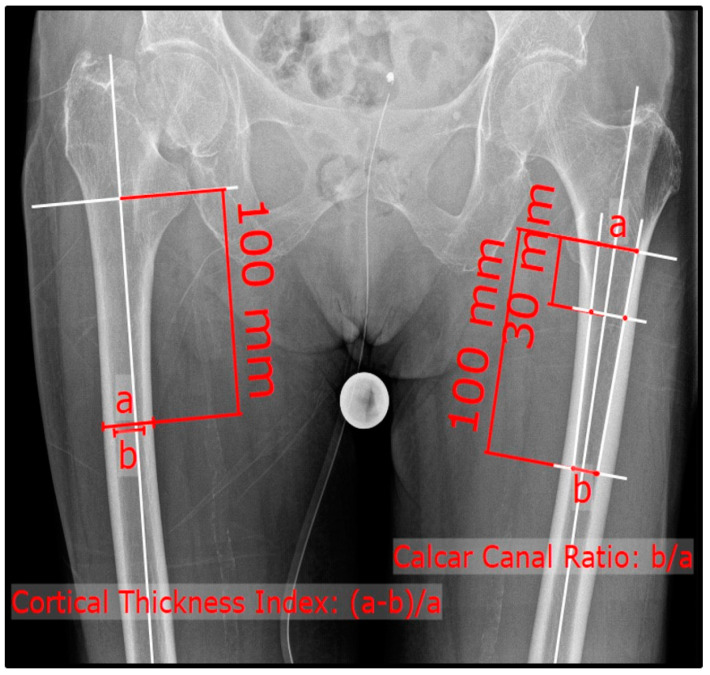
Antero-posterior X-ray of the pelvis; formula for the cortical thickness index (right) and canal calcar ratio (left).
